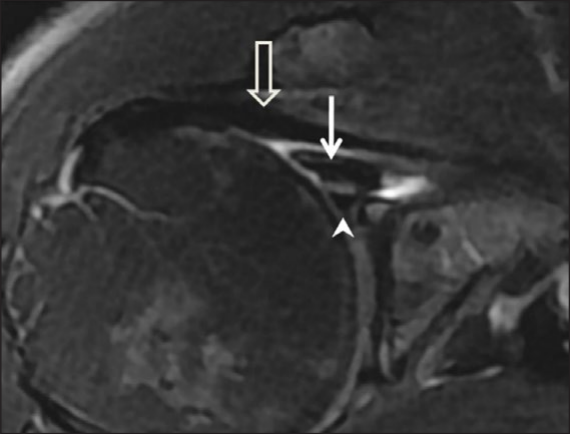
Cord-like middle glenohumeral ligament. Axial fat saturated T2-weighted MR image depicts a thick cord-like middle glenohumeral ligament (arrow). Subscapularis tendon (open arrow) and anterior labrum (arrowhead) are also shown on this section.
